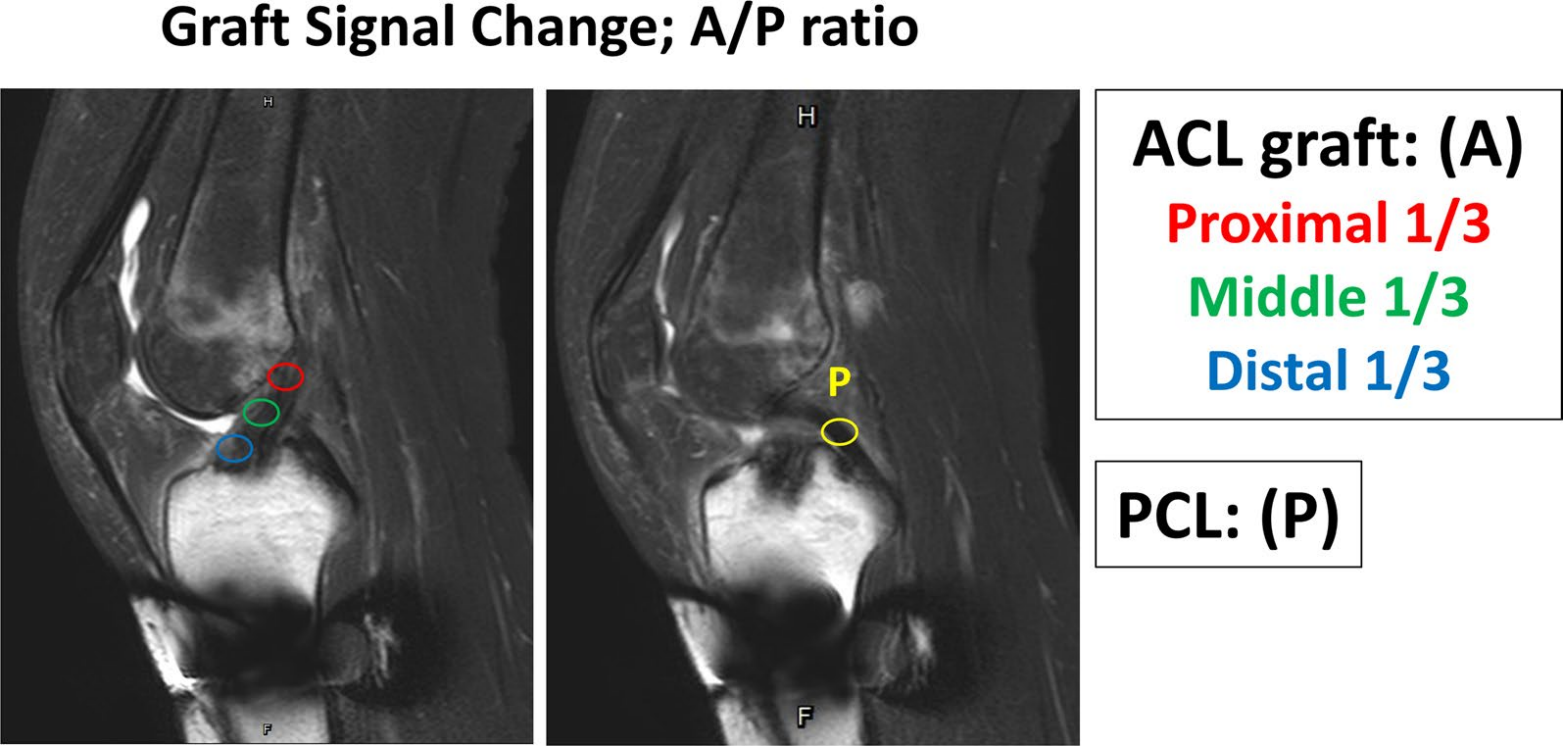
The graft maturation was recorded as graft signal change detected from the T2 sagittal view in MRI. The whole graft signal (proximal, middle, and distal portions of the ACL graft) was averaged and normalised by individual PCL signal (A/P ratio)
